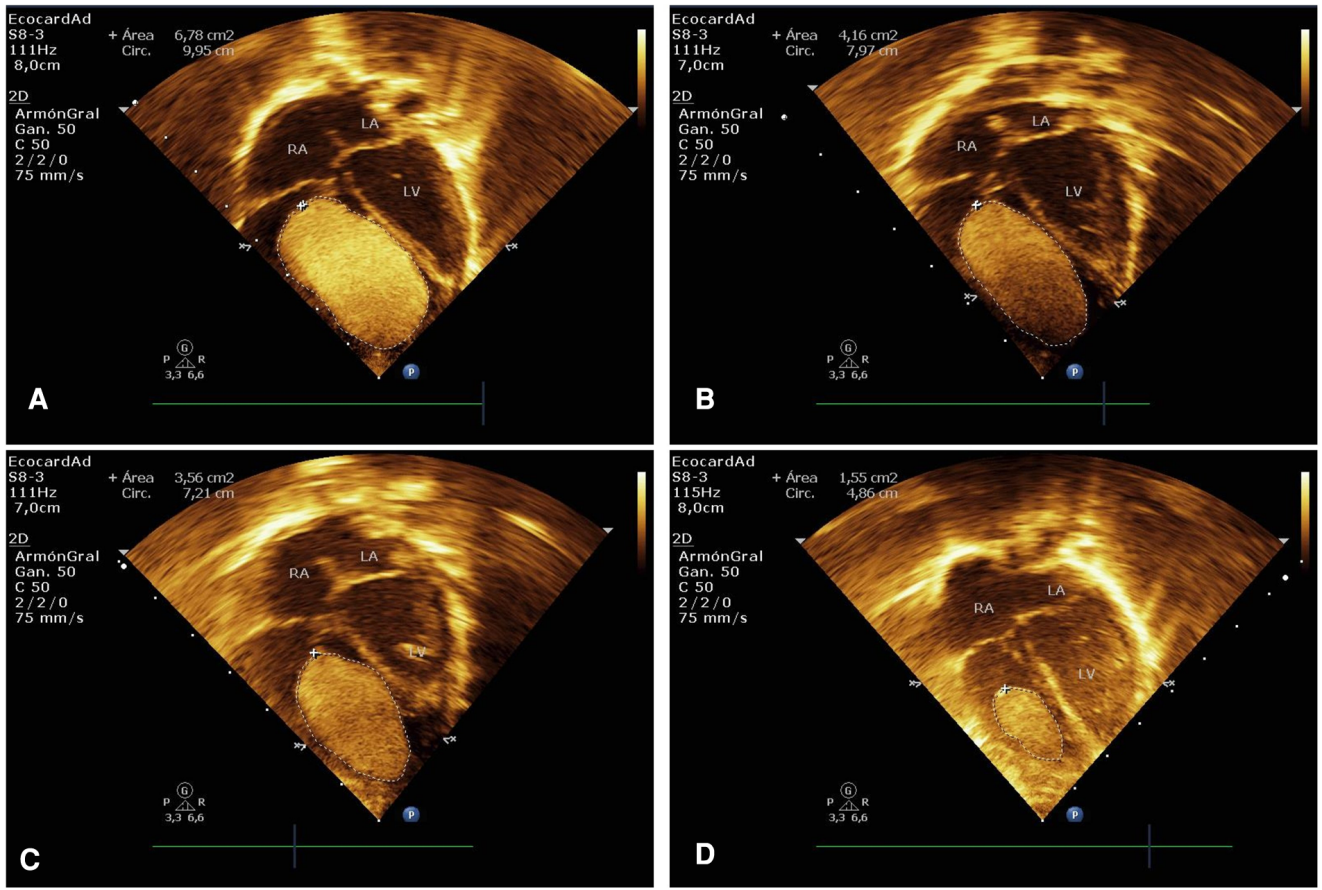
Case 1. (A) Immediate postnatal transthoracic echocardiogram in apical 4-chamber view showing a large hyperechogenic and homogeneous mass of 37 mm × 20.7 mm in size (area of 6.78 cm^2^), which occupies a large part of the right ventricular cavity, compatible with a giant rhabdomyoma. (B–D) Echocardiographic control at 2, 3, and 13 weeks after treatment with everolimus showed a significant reduction in the size of the rhabdomyoma.
